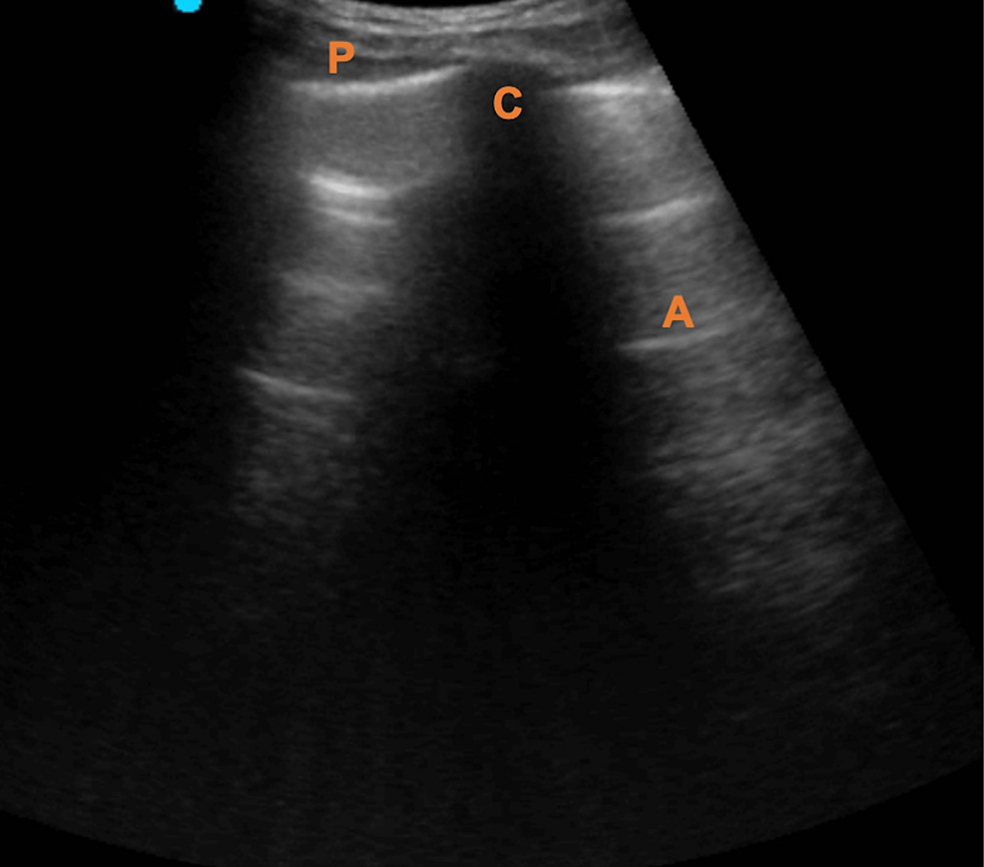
Assessment of the left apex position using the lung ultrasound. P: pleura; A: A lines; C: rib (and acoustic shadow).
